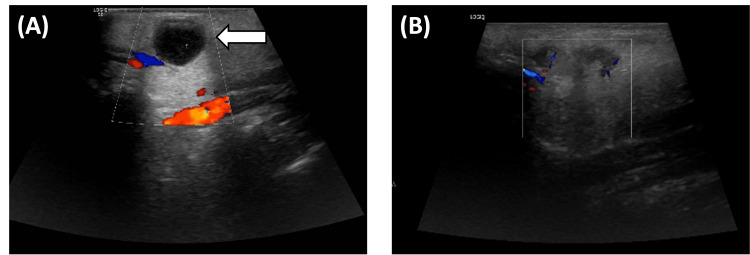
Ultrasound evaluation of the left parotid region pre and post-biopsy (A) Pre-biopsy ultrasound image of the left parotid gland, showing a well-defined, vascularized lesion within the superficial lobe (arrow). (B) Post-biopsy ultrasound image demonstrating postoperative changes, with a hypoechoic area at the site of the excised lesion. No residual or recurrent mass lesions are observed, and surrounding soft tissues in the neck region appear unremarkable, indicating no significant postoperative complications.
